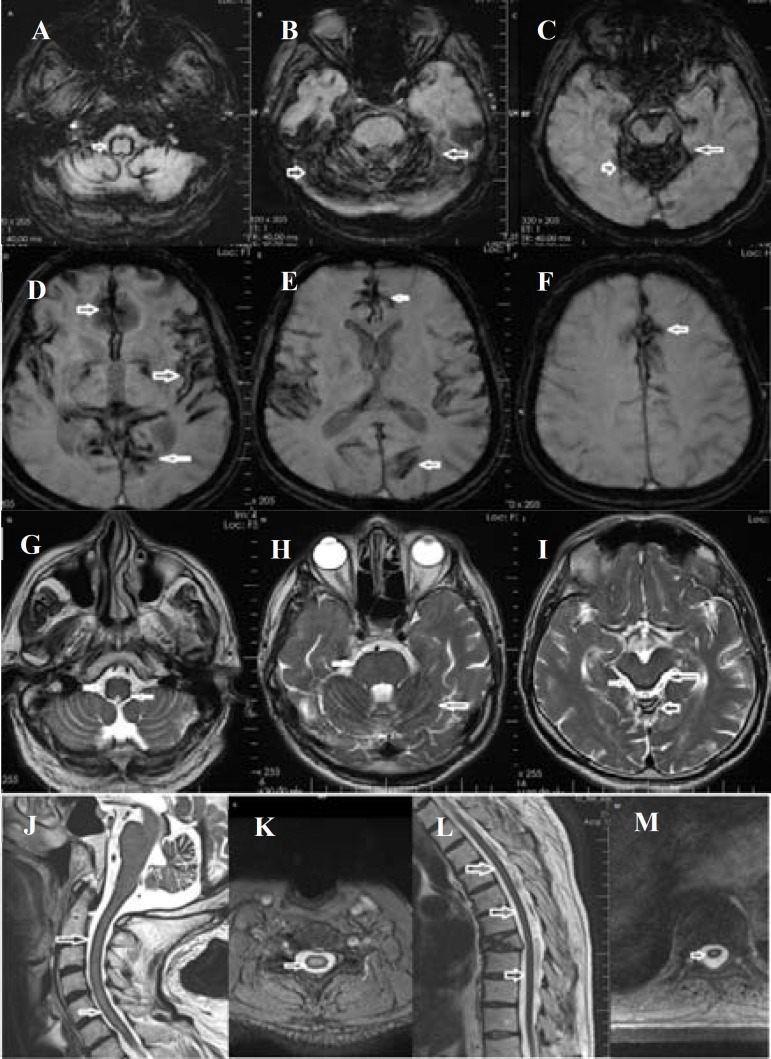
Brain magnetic resonance imaging. Susceptibility (A-F) and T2-weighted imaging (G-I) show hemosiderin deposition especially on brainstem, cerebellum (showed with white arrow). T2-weighted imaging reveals the hemosiderin deposition as hypointensity around the spinal cord as well (J-M).
